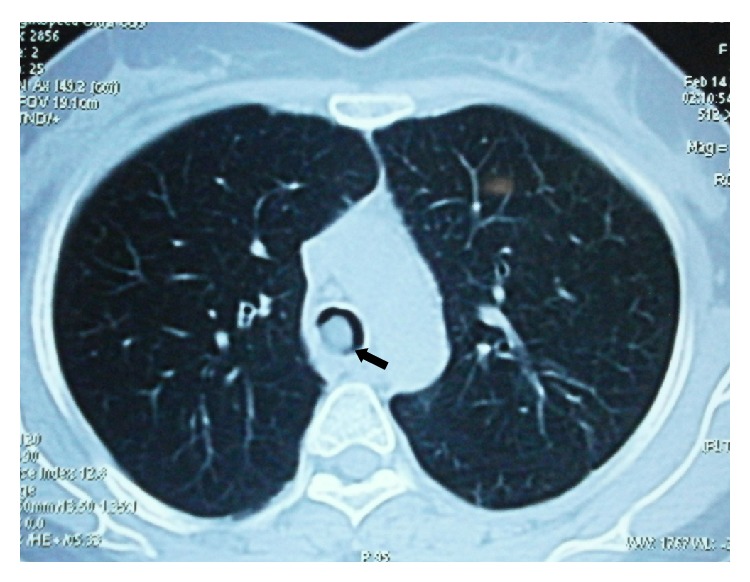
CT scan of the thorax showing a endoluminal polypoidal growth (arrow) arising from the posterior wall of the tracheal bifurcation.
